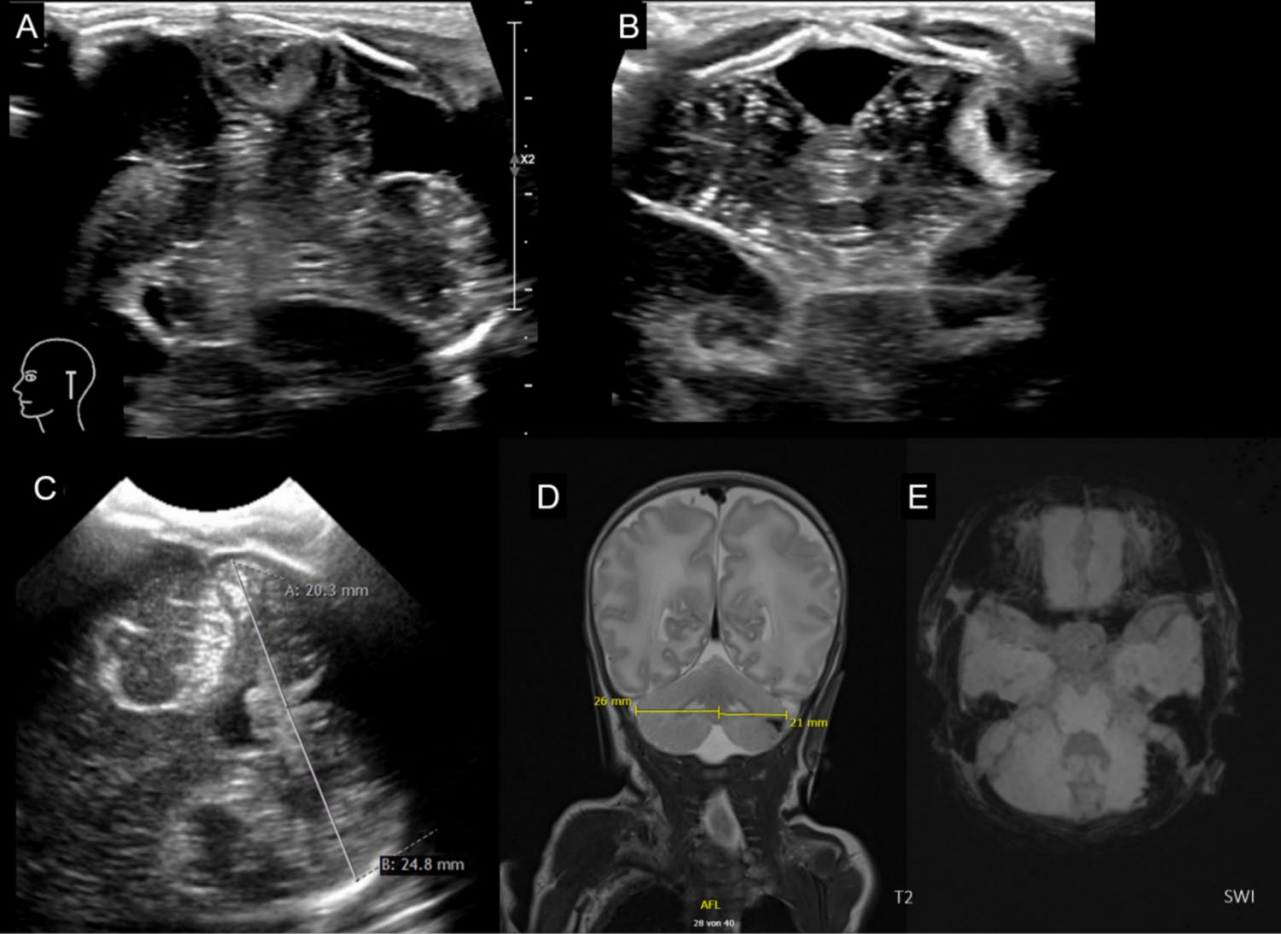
Limited cerebellar hemorrhage (CBH) left posterior-lateral hemisphere in a preterm infant of 29 weeks gestational age. A Coronal ultrasound scan through the mastoid fontanel, B corresponding transnuchal ultrasound scan on the fourth day of life shows the limited CBH (< 1/3 of the left hemisphere). C On the follow-up scan at postmenstrual age (PMA) of 34 5/7 weeks through the left mastoid fontanel, an asymmetrical appearance with smaller left hemisphere can be identified. D T2 weighted MRI scan at PMA 36 6/7 weeks and susceptibility weighted imaging (SWI) confirm the asymmetry with a hypoplastic left hemisphere and residual hemosiderin deposition after the CBH
